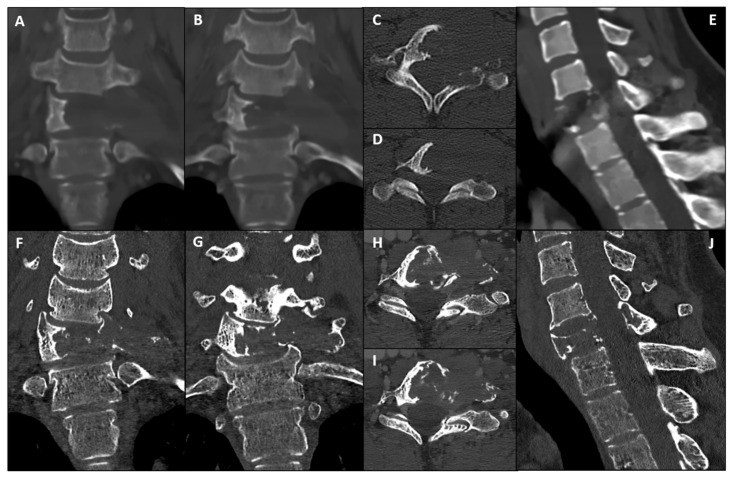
CT radiographic evolution under Neoadjuvant Denosumab Therapy. (A–E) Baseline assessment. Standard bone-window CT reconstructions (Coronal (A,B), Axial (C,D), Sagittal (E)) at diagnosis, showing a massive, purely osteolytic Giant Cell Tumor involving the C7 vertebral body and posterior elements. Note the voluminous left paravertebral exophytic component, which extends significantly into the soft tissues. (F–J) Twelve-month follow-up (Photon-Counting CT). Corresponding Coronal (F,G), Axial (H,I), and Sagittal (J) views acquired via Photon-Counting Detector CT following Denosumab treatment. Comparison: While the images confirm a positive biological response—evidenced by tumor shrinkage and the formation of a hyperdense sclerotic rim (enhanced visualization due to PCCT resolution)—they simultaneously highlight the progressive mechanical failure of the anterior column. Note the development of a severe right-convex cervicothoracic scoliosis (left lateral bending) centered at the C7 fulcrum.
